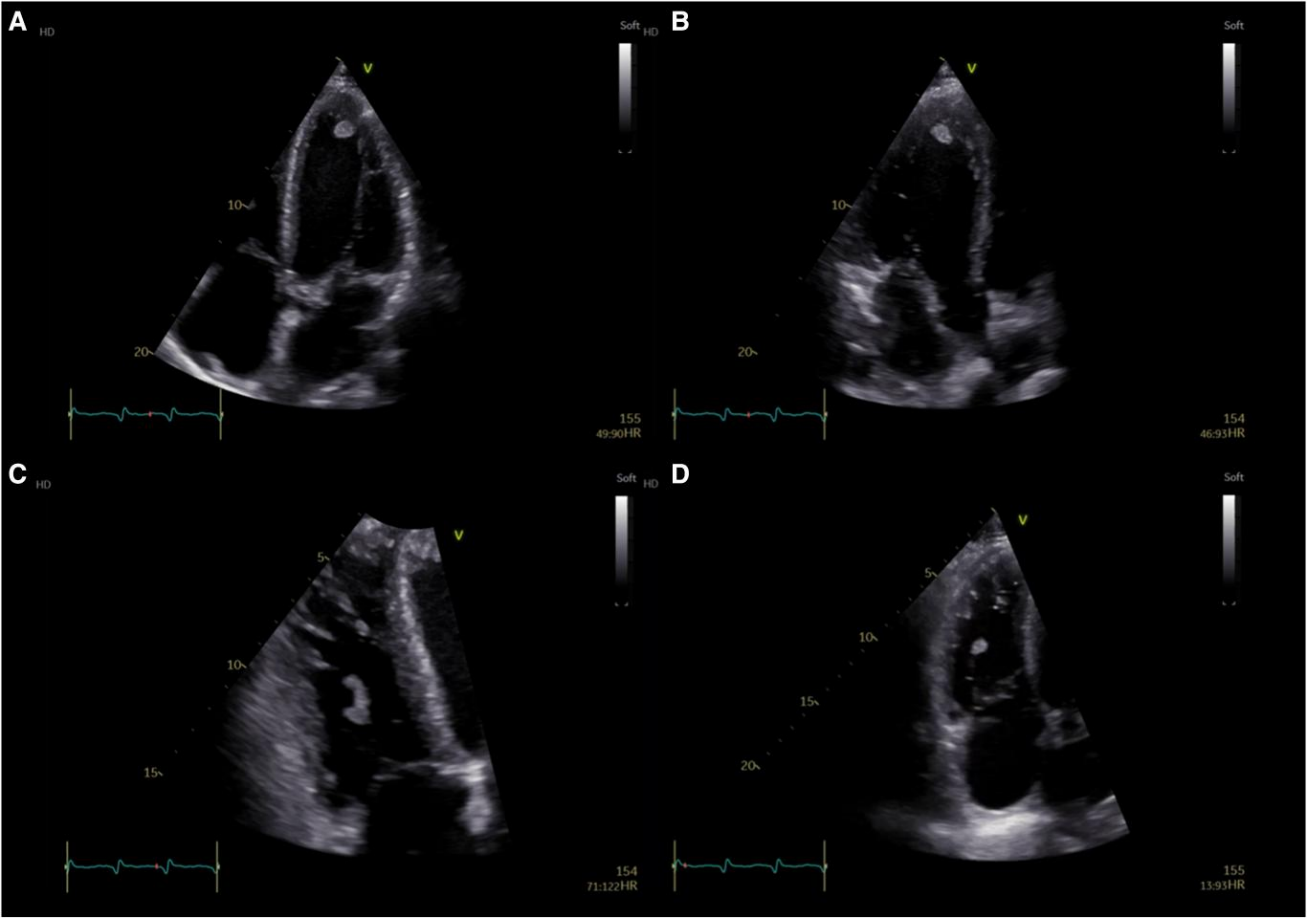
Echocardiography revealing two masses. A left ventricular mass at the apex is seen in apical four-chamber (*A*) and apical three-chamber views (*B*). The second mass is observed on the tricuspid valve chordae (*C* and *D*).
